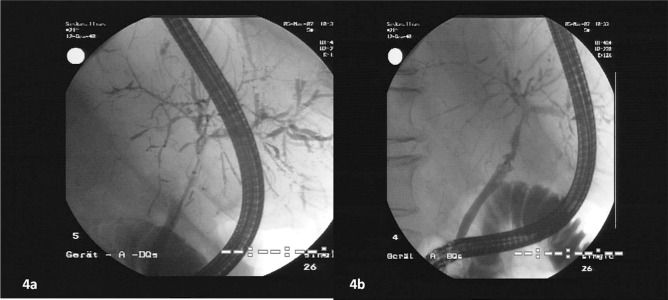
Figure 4a Demonstrates ERCP Images of a Patient With ITBL Who was Diagnosed Only on Ultrasound. ERCP Missed the Diagnosis at This Time. Figure 4b is The Same Patient Seven Months Later. By The Second Examination the Diagnosis ITBL Was Also Confirmed by ERCP.
